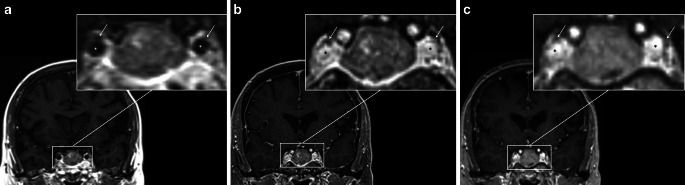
Contrast-enhanced, multi-dynamic-multi-echo-based (a); Volumetric Interpolated Brain Examination (VIBE)-based (b); and Magnetization Prepared Rapid Acquisition Gradient Echo (MPRAGE)-based (c) T1-weighted imaging data (*coronal plane*) depict parasellar anatomy comparably in a female patient, 22 years of age at the time of MRI (non-invasive, lactotroph adenoma). The left and right oculomotor nerve (*arrows*) is detectable on each sequence acquisition. Notice the differences in carotid artery enhancement (*asterisk*), while the pituitary adenoma enhances in a relatively stable manner, despite protocol-related delays (Table 2)
